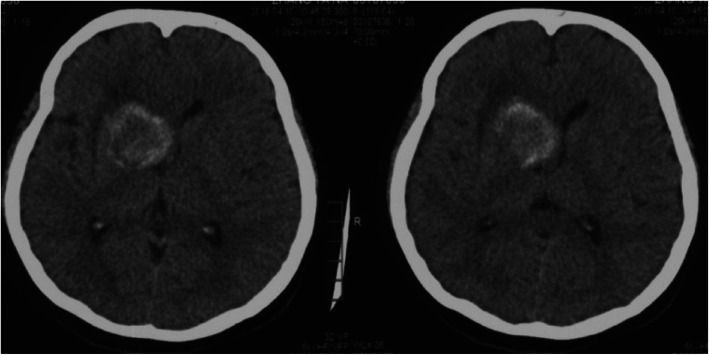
Preoperative CT scan. It showed an occupancy with hemorrhage
